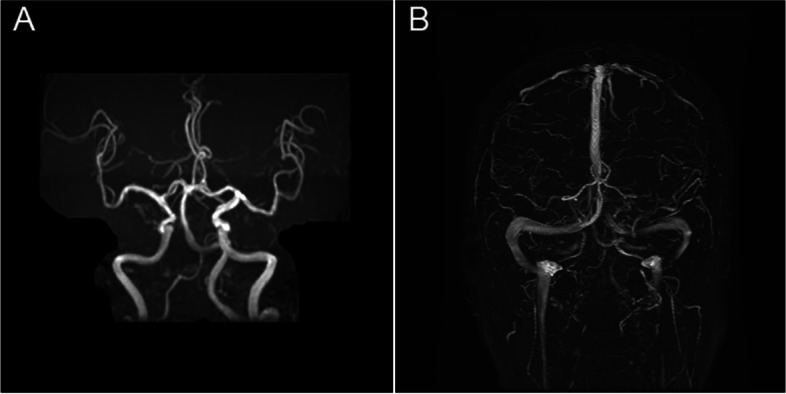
Cerebral MRI findings. A MRA shows mild atherosclerotic changes. B MRV is normal
